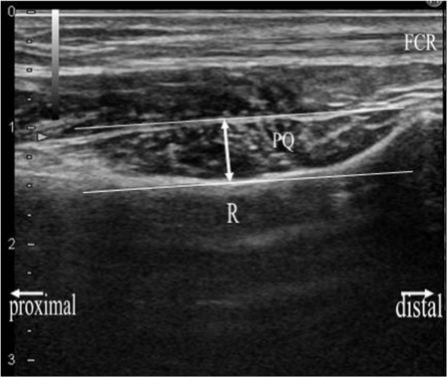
Sonographic longitudinal image of the examined part of the distal forearm, including anatomical reference and measurements of PQ thickness, using a 17-MHz transducer. Left hand of a 33-year-old healthy male volunteer. We referred to the line tangential to the volar concave aspect of the radius. FCR: flexor carpi radialis; PQ: pronator quadratus; R: radius; full-lined arrow: maximum thickness of the PQ.
